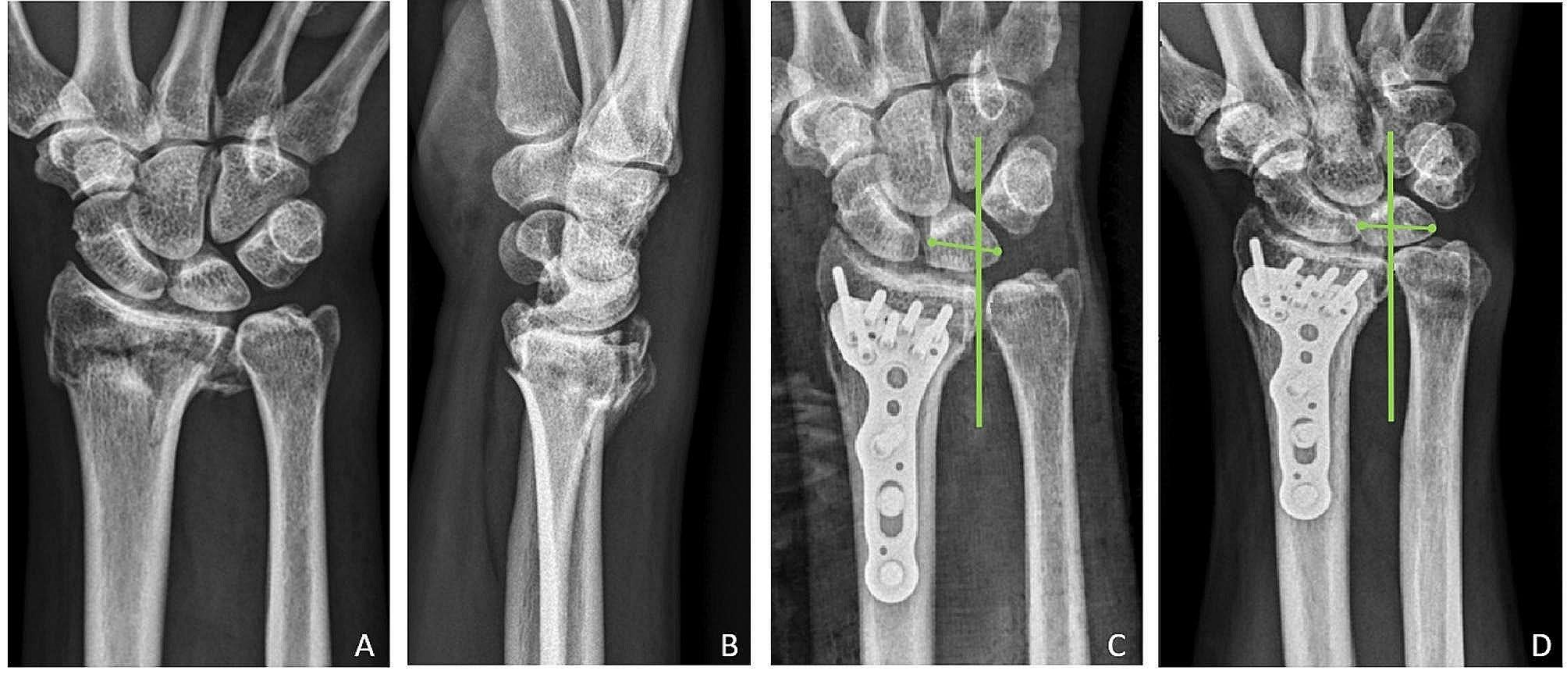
A 61-year-old man with right distal radius fracture. Radiographs showing (A) and (B) the fracture before treatment. (C) Radiograph after open reduction and internal fixation. (D) Ulnar carpal translation (UCT) was observed in the 3-month postoperative radiographs
